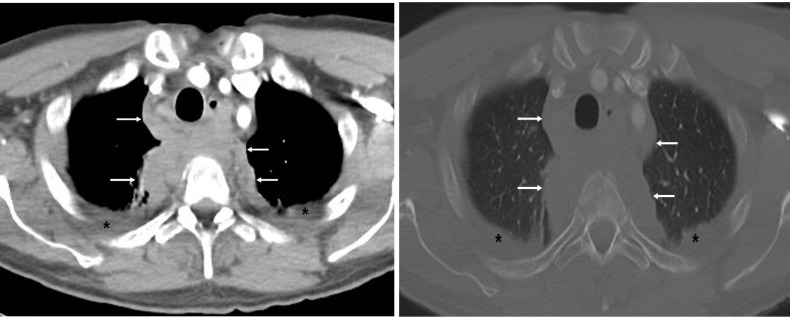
Mediastinal (first image) and bone (second image) windows of contrast enhanced chest CT of a 26 years old male with multiple thoracic vertebral fractures sustained after a high speed road traffic accident show a large para-vertebral high tissue density (arrows) suggesting mediastinal hematoma. Esophagus is displaced anteriorly to the left side of the trachea by the hematoma. Bilateral pleural effusion (asterisk) is also present. Vertebral fractures are not visible on these images
